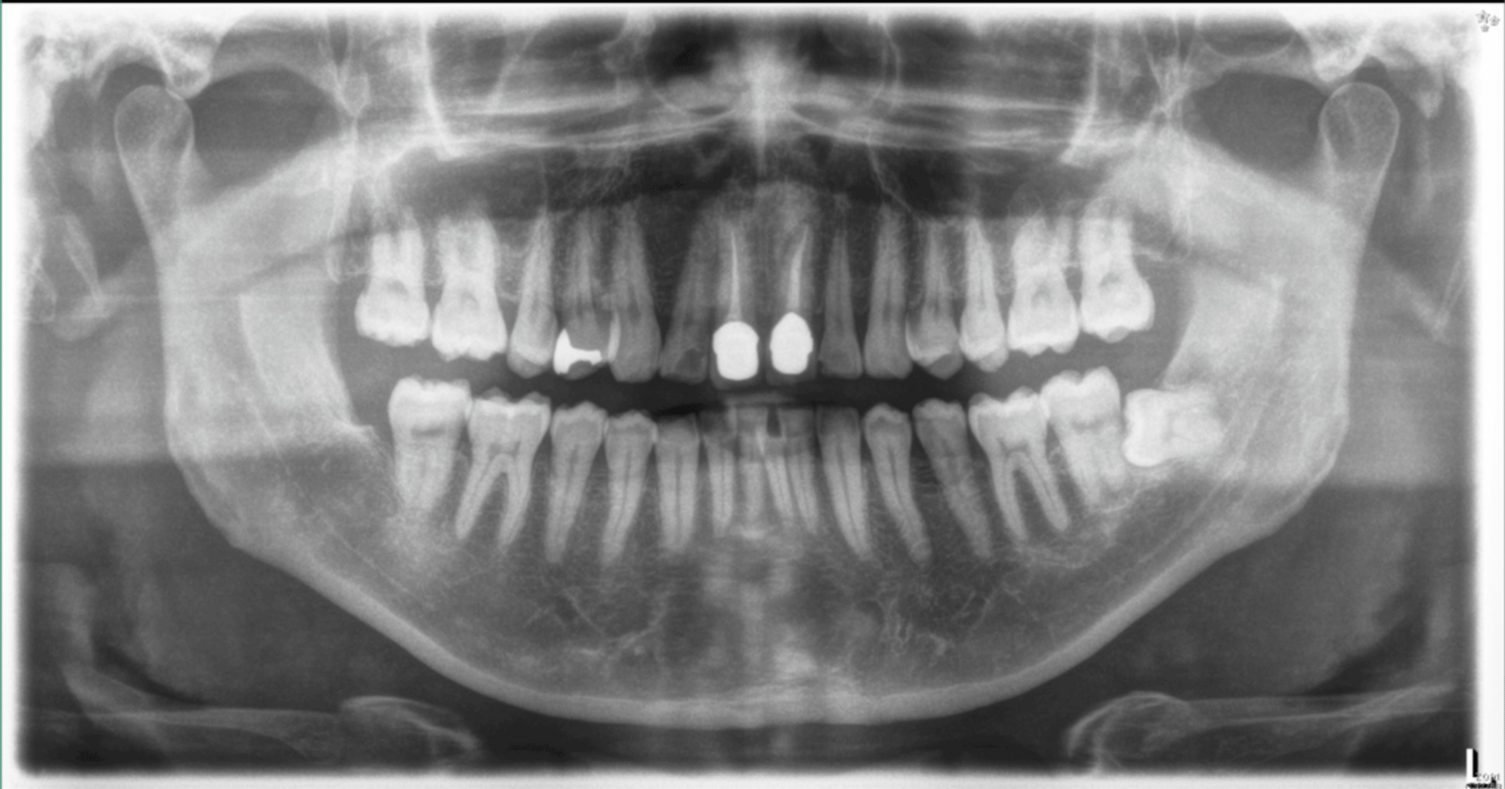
OPG showing no apparent abnormalities OPG: orthopantomography
